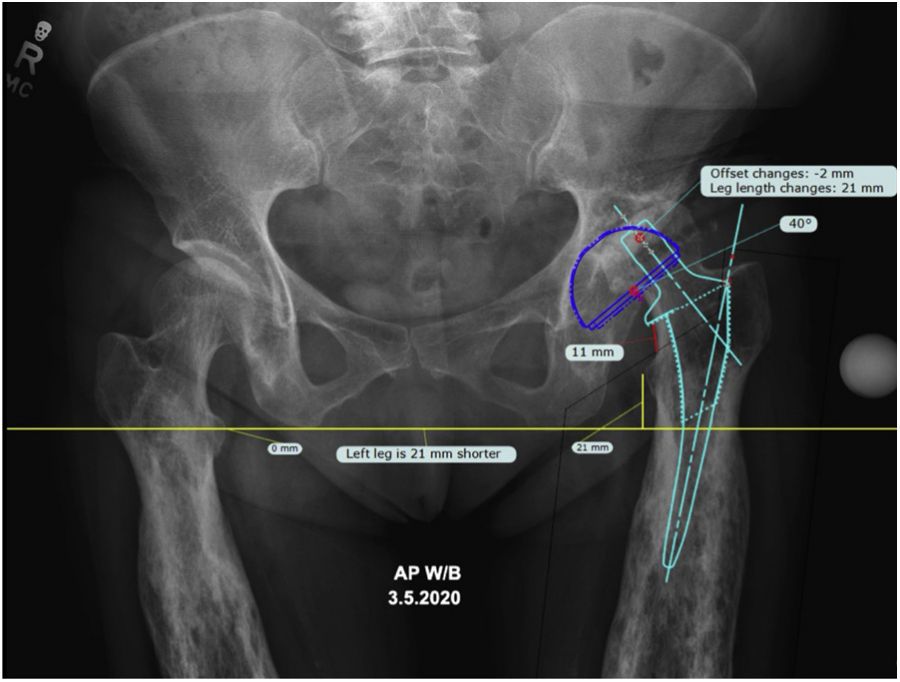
Preoperative anteroposterior pelvis radiograph with templating using the TraumaCad-software system (Voyant Health) demonstrating a 21-mm leg length discrepancy.
